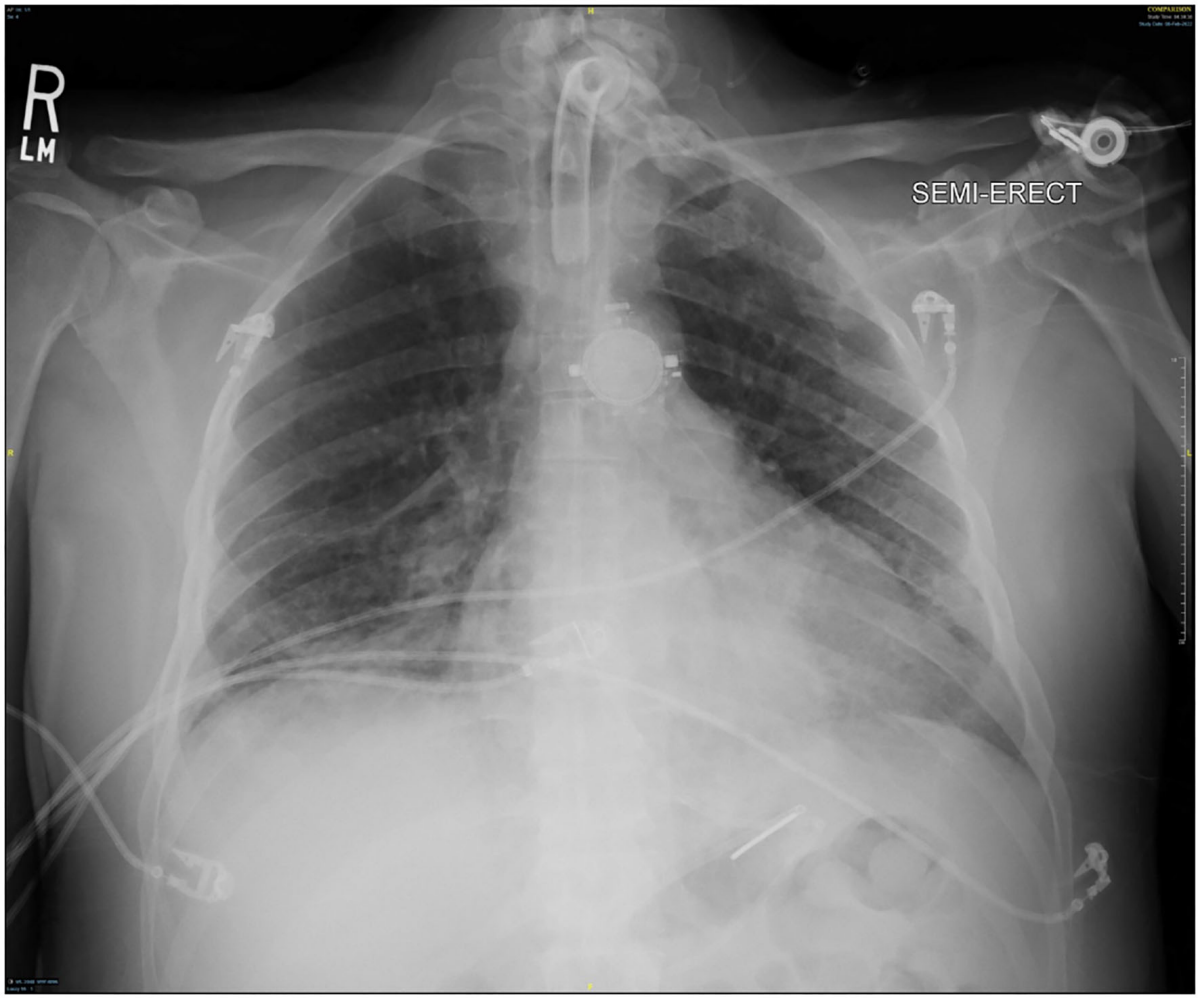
Chest x-ray after ECMO decannulation.
